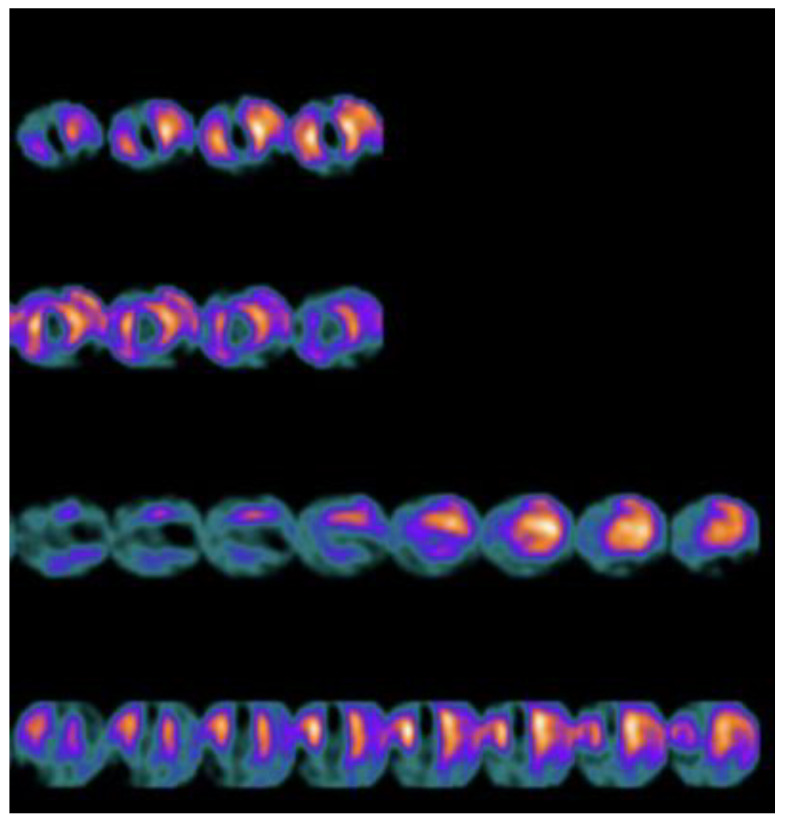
123I-meta-iodobenzylguanidine (123I-MIBG) single-photon emission computed tomography (SPECT) in a patient with previous myocardial infarction of the apex, with involvement of the anterior and the posterior wall (absent uptake of the radiopharmaceutical in these sites).
